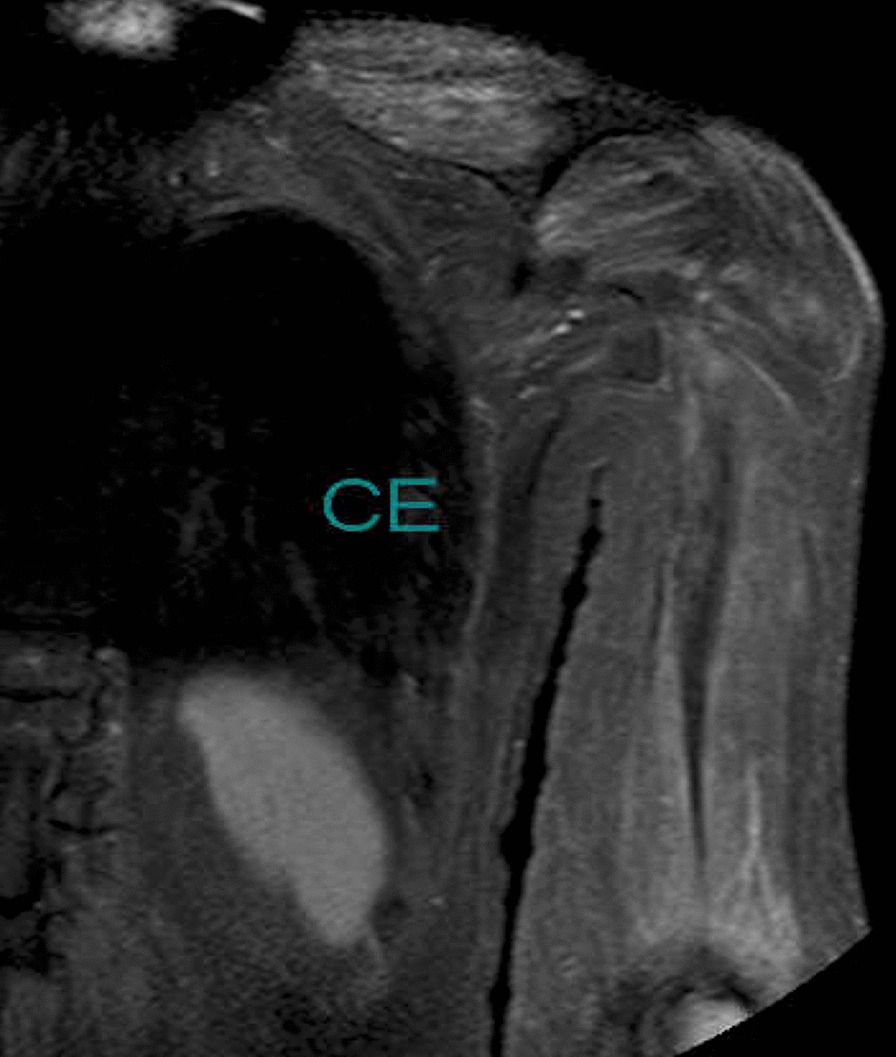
Brachial contrast-enhanced magnetic resonance imaging demonstrated hyperintense muscles in T2-weighted sequences
